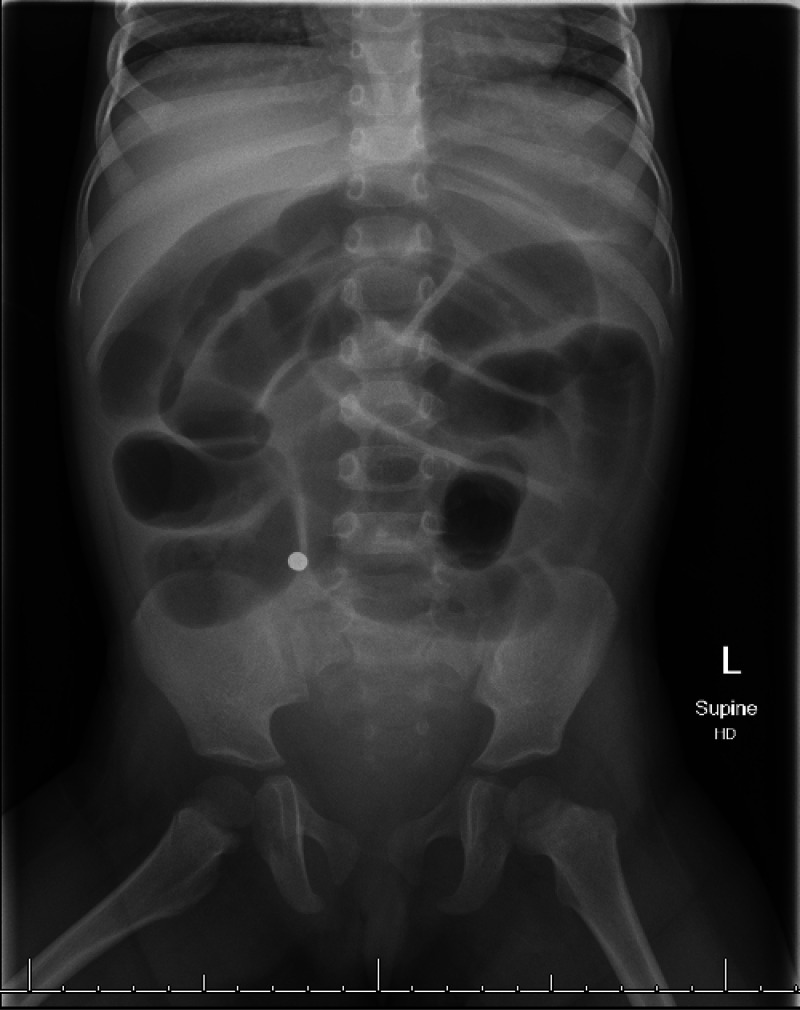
X-ray abdomen (frontal view), single rare earth magnet
